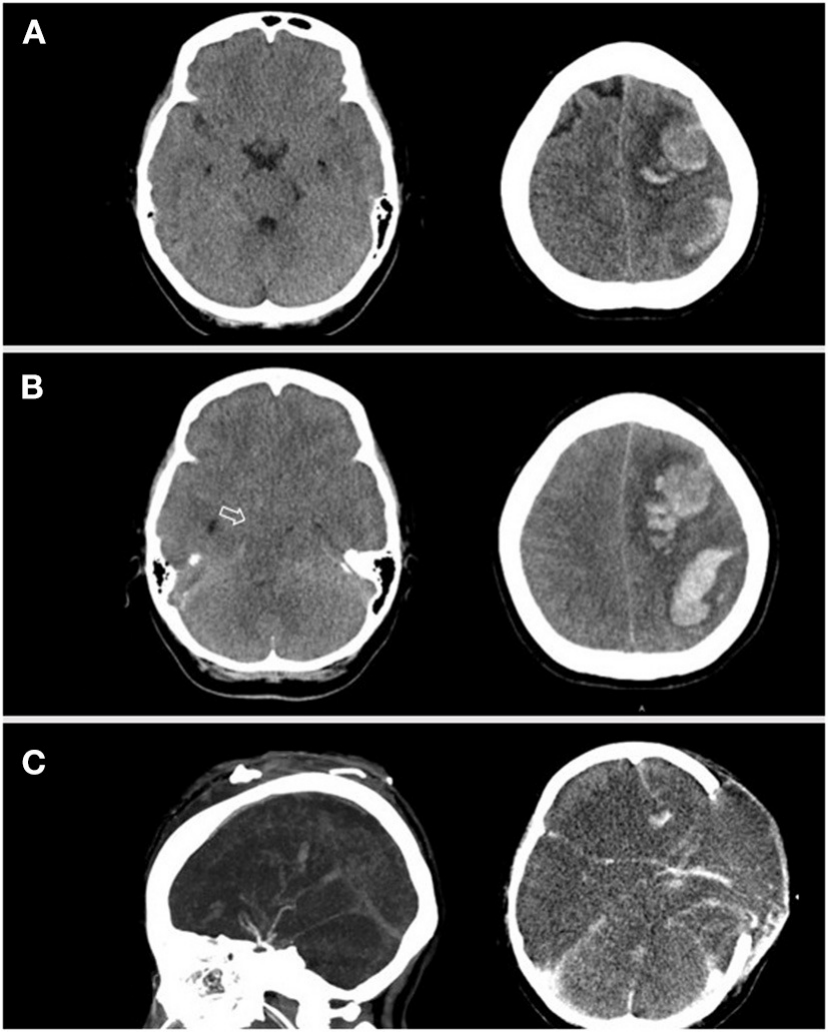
A series of brain CT over the disease course. (A) Brain CT revealed two lobar hemorrhages located in the left frontal and parietal lobes with perifocal edema. (B) Five hours later, the patient's brainstem reflex was lost, and brain CT showed progressive hemorrhage with diffuse cerebral edema and bilateral uncal herniation (arrow). (C) Contrast-enhanced brain CT obtained 5 days after craniectomy showed suboptimal vascular image quality due to prominent intracranial hypertension.
